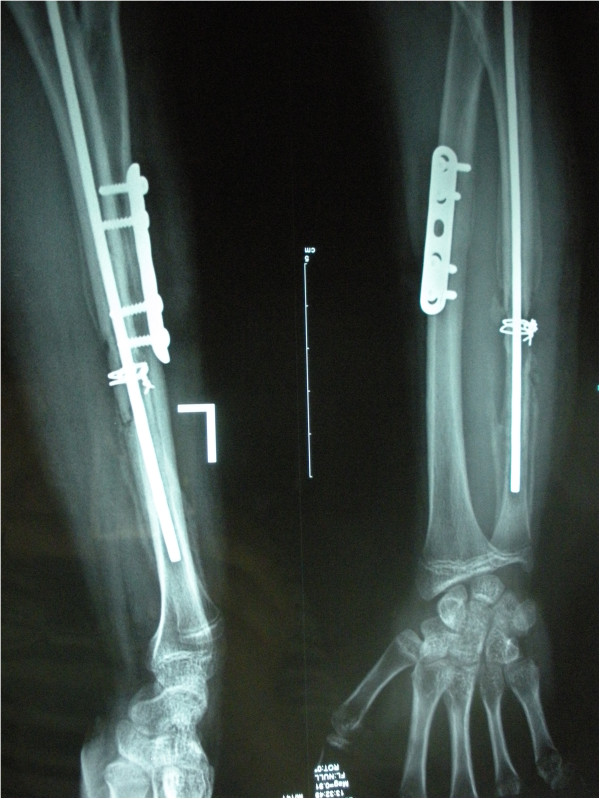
Radiograph of a 15-year-old man (case 5) who sustained comminuted fractures of ulna and radius had an infected ulna nonunion.
